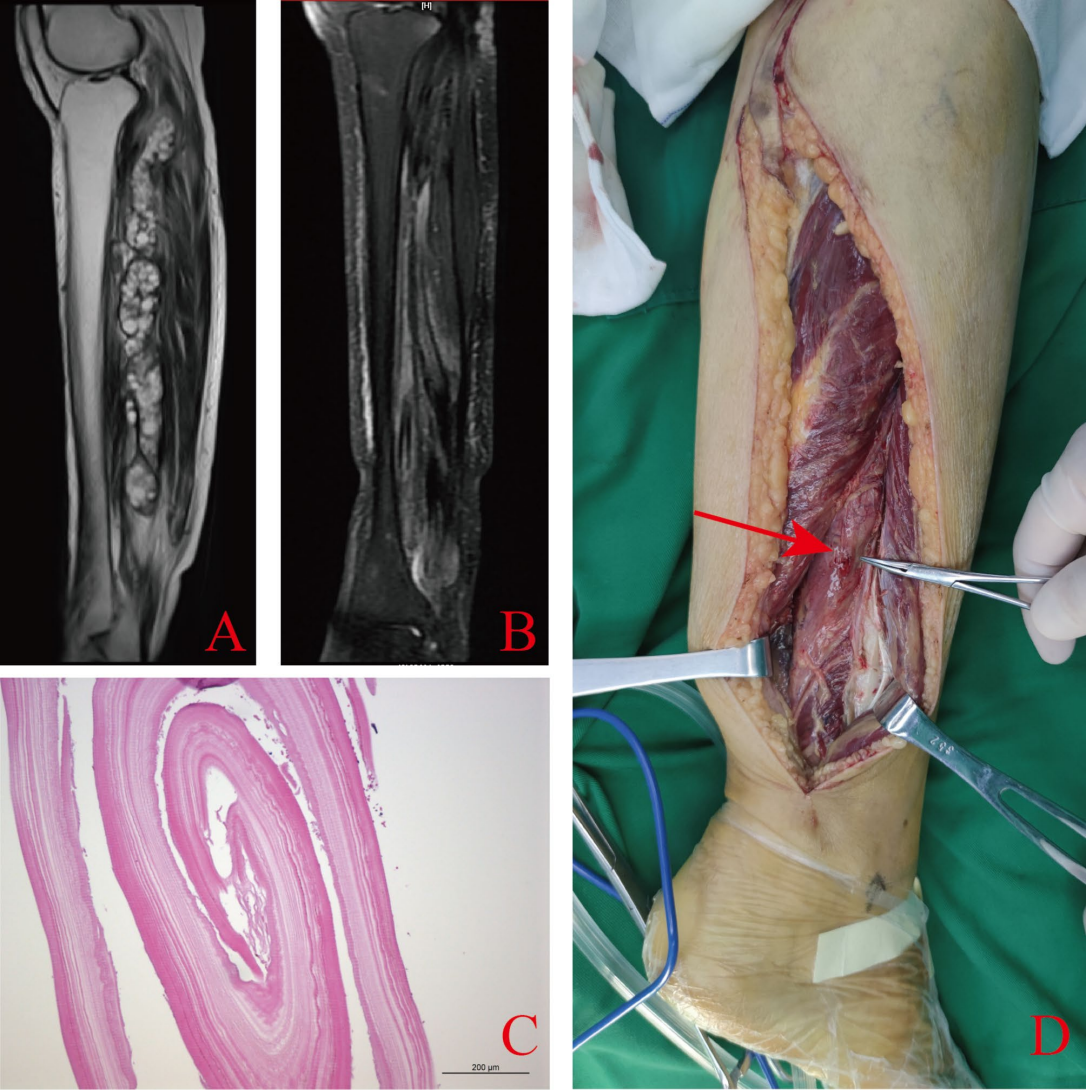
Information regarding the patient’s muscle hydatid cyst. (A) MRI of right lower leg at initial presentation. (B) MRI of the patient’s right lower leg one year after surgery. (C) Pathological section of right calf muscle hydatid cyst. (D) Intraoperative picture: muscle hydatid cyst pointed by red arrow
